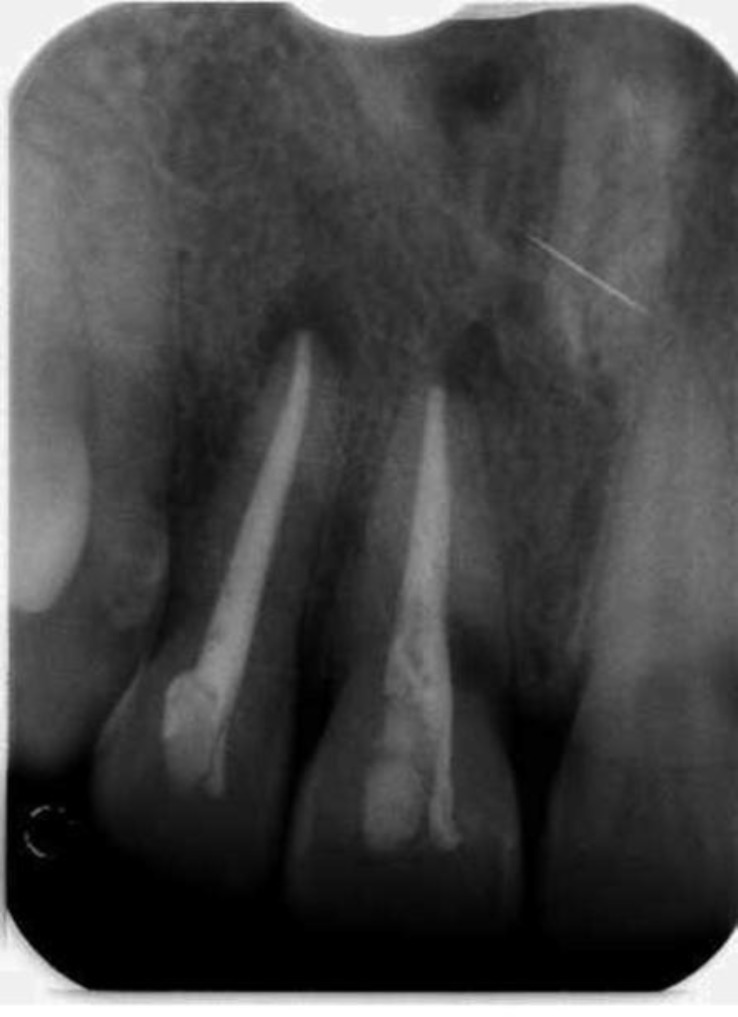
Immediate post-op Radiograph
